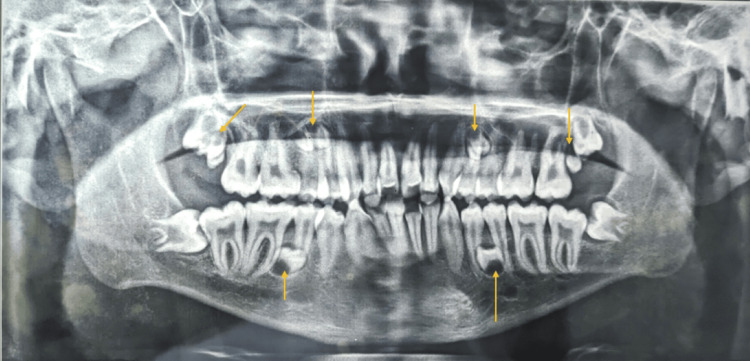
Pretreatment OPG (orthopantomogram) with parapremolar and paramolar in respective quadrants.
